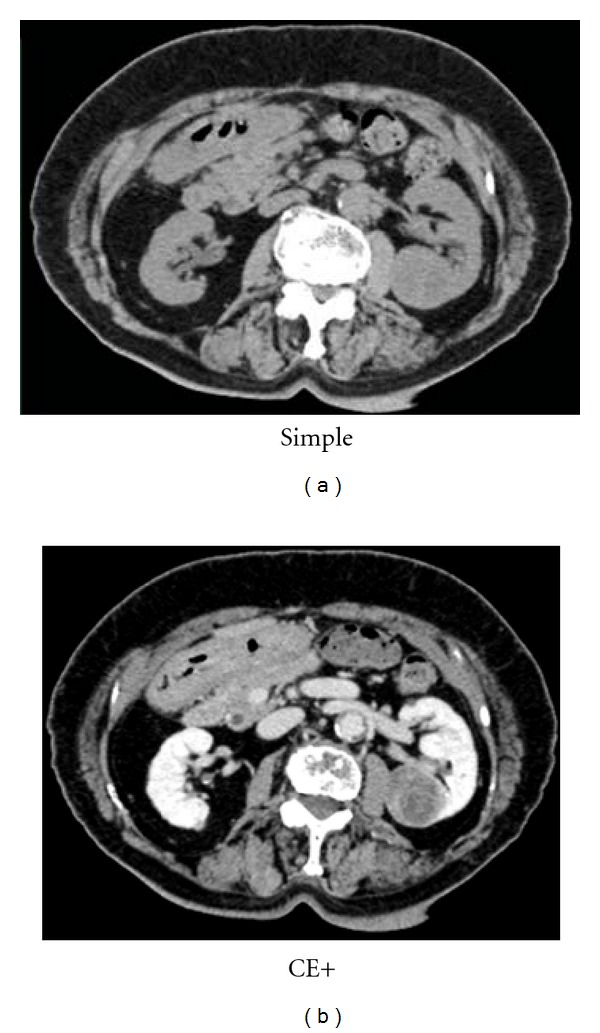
Computed tomography at the time of diagnosis. It showed a heterogeneously enhanced left renal mass 35 mm in diameter.
